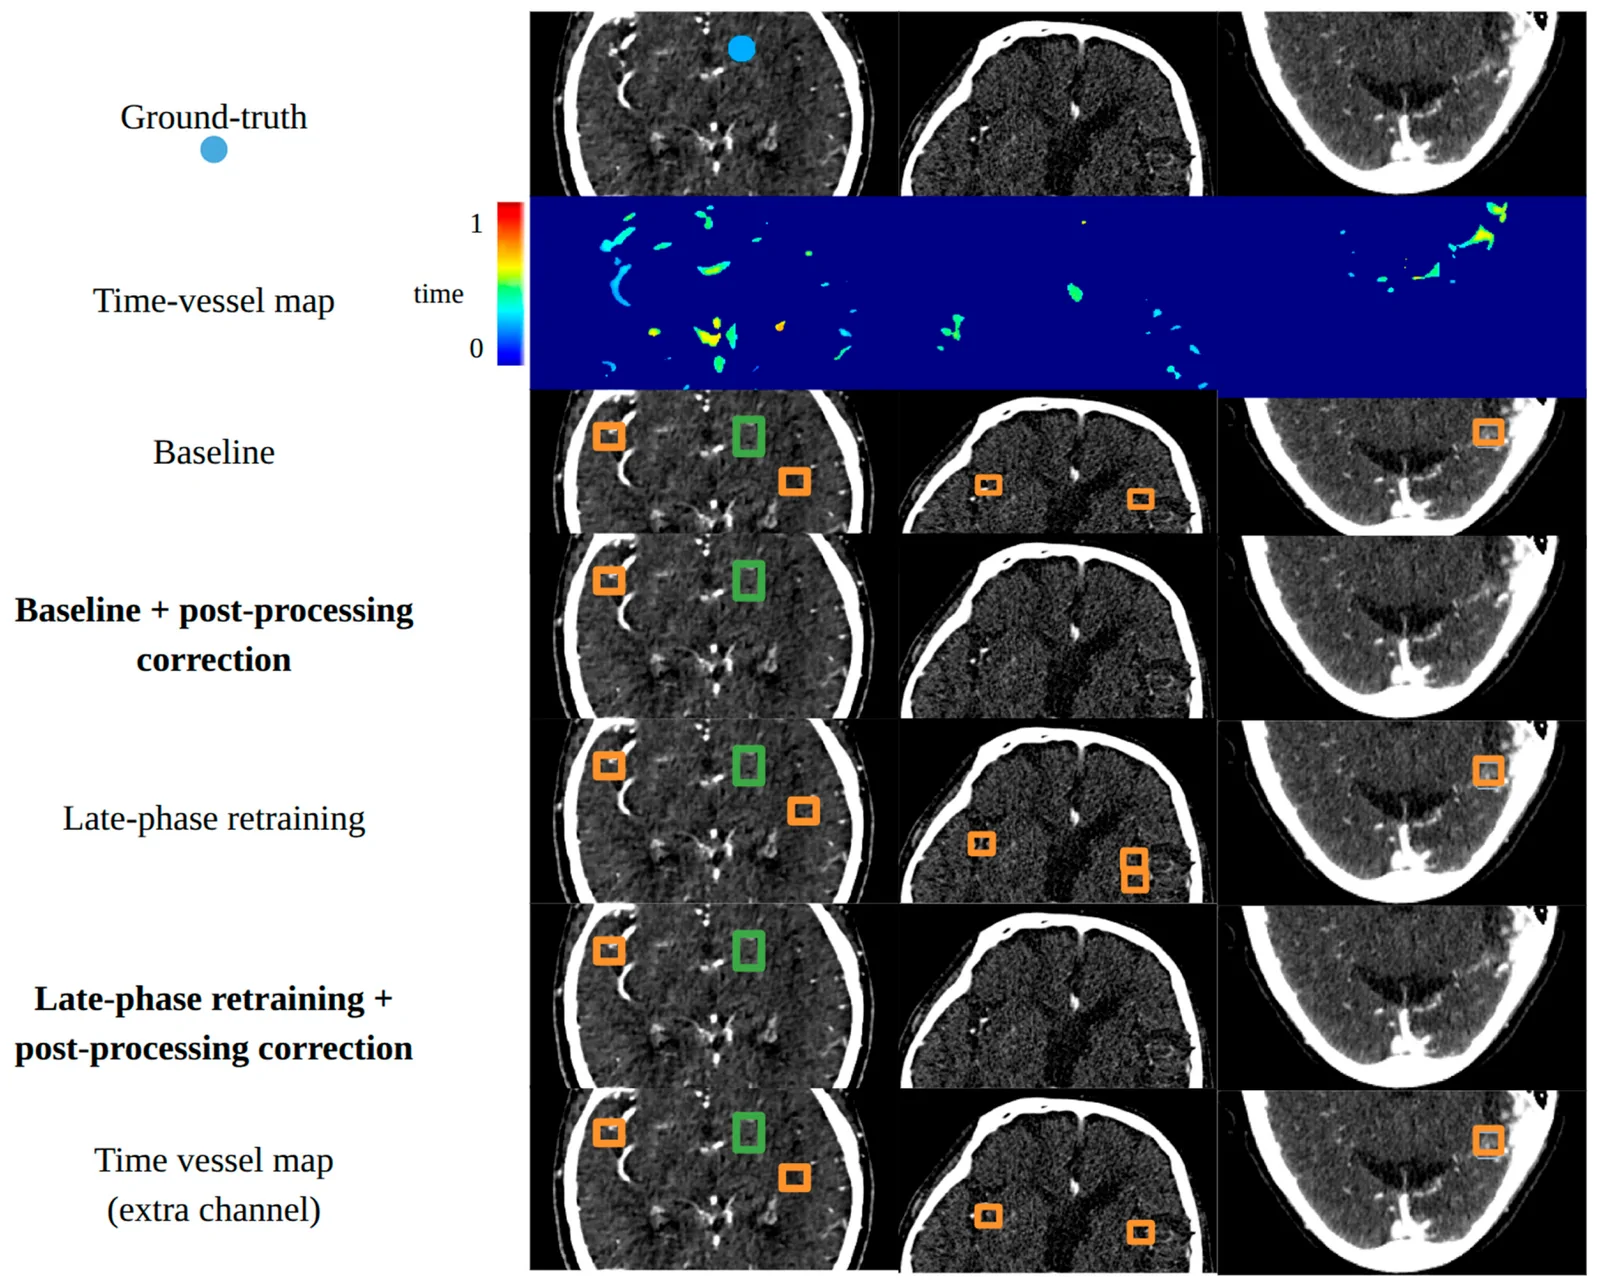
Qualitative results on the external test cohort, for the benchmarked methods, including estimated time-vessel maps with the CTA-to-time-vessel map generator. Blue blobs denote occlusion annotations; orange boxes denote incorrect occlusion detections; green boxes denote true occlusion detections. Methods in bold use the post-processing correction framework.
